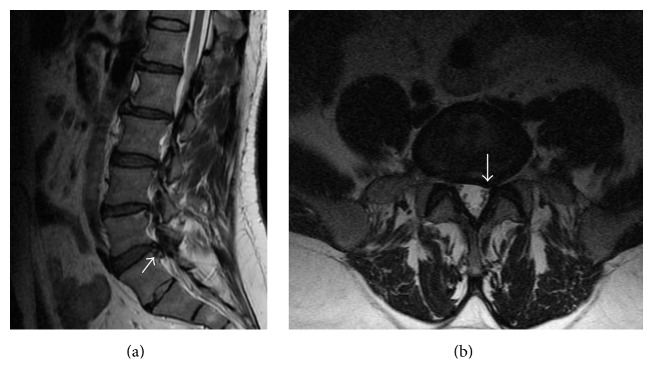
An extruded disc herniation that slightly migrated downward at L5-S1 level on T2 weighted sagittal (a) and axial (b) MR images after 3 years.
